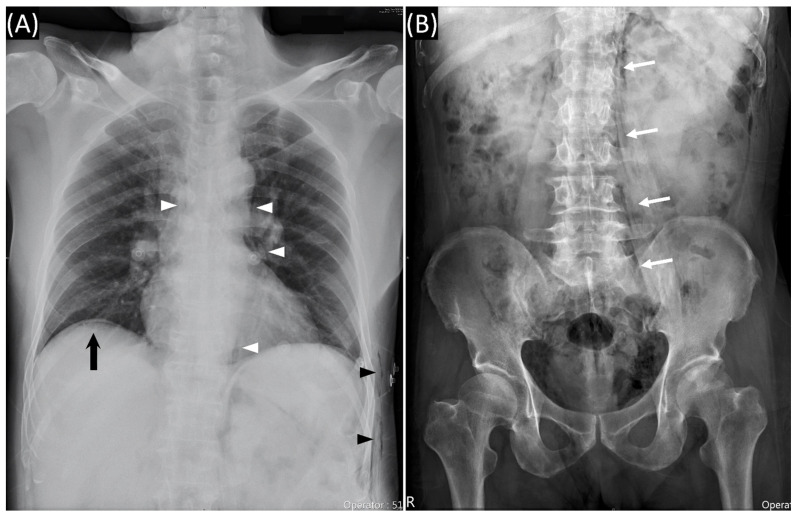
(A) Chest radiography showed subcutaneous emphysema (black arrowheads), pneumomediastinum (white arrowheads), and subdiaphragmatic free air (black arrow). (B) Abdominal radiography showed paraspinal air which outlines the psoas muscle (white arrows).
